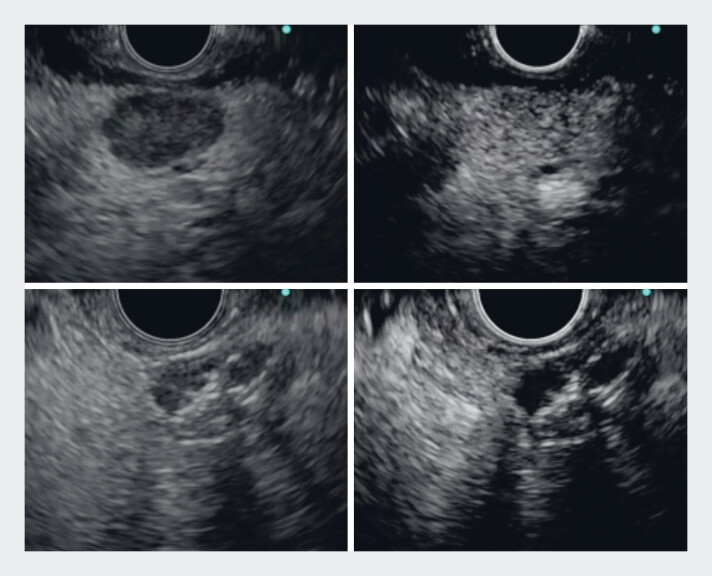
Contrast-enhanced endoscopic ultrasound (CE-EUS) findings before and after endoscopic
